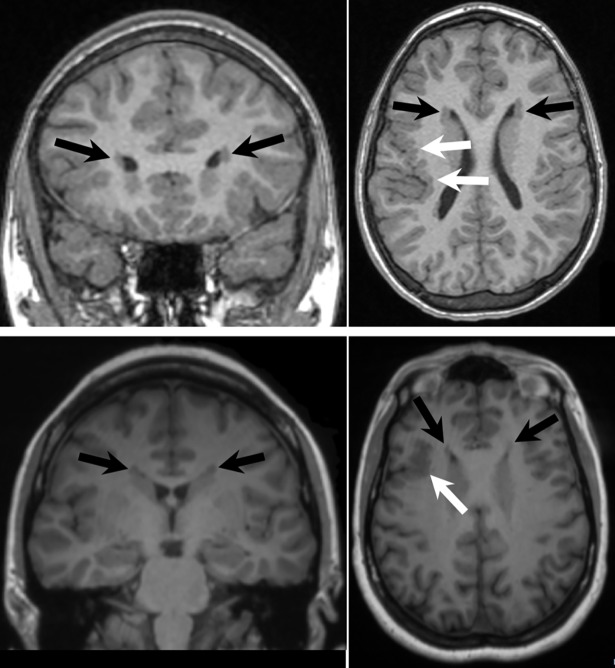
Brain MRI of subjects pvhnz9000cfc1 (top) and mother (bottom). Images are coronal T1-weighted (left column) and axial T1-weighted (right column). The images all show bilateral periventricular nodular grey matter heterotopia maximal in the frontal regions (black arrows). The axial images show over-folded cortex in the deep perisylvian/insular region on the right consistent with polymicrogyria (white arrows).
